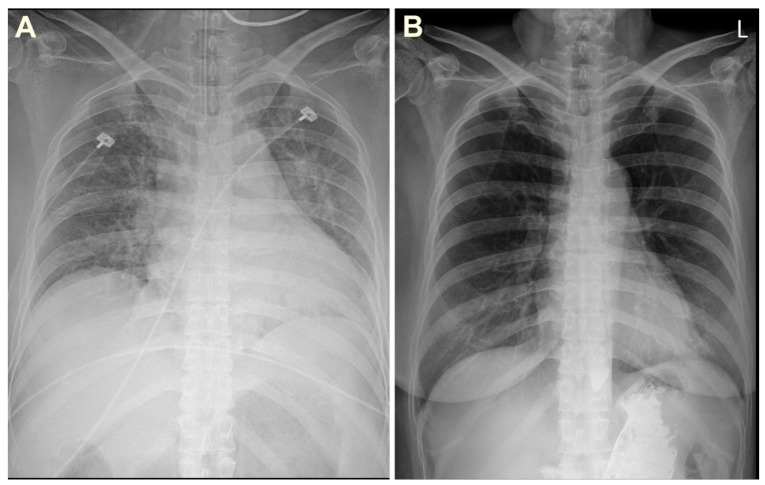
Chest radiographs on admission (A) and discharge (B).
